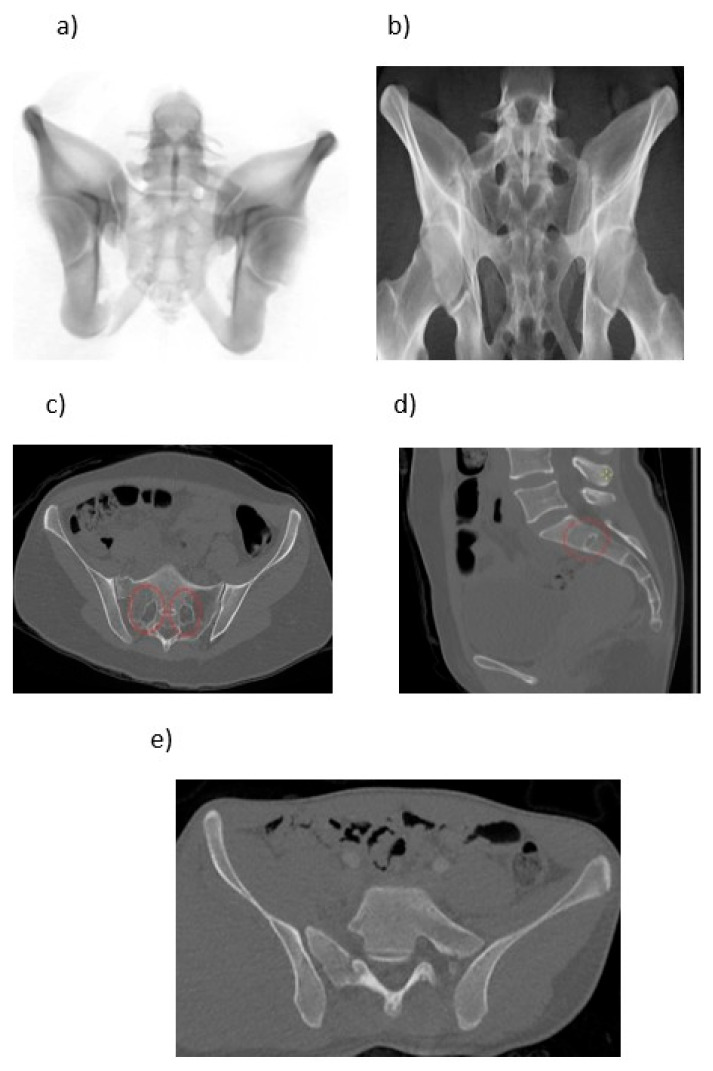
Qualitative radiographic features of sacral dysmorphism on pelvic outlet and CT images. (a) Crest–disc colinearity, (b) oblique alar transverse process, (c) noncircular S1 foramina, (d) S1–S2 residual disc, and (e) steep alar slope.
